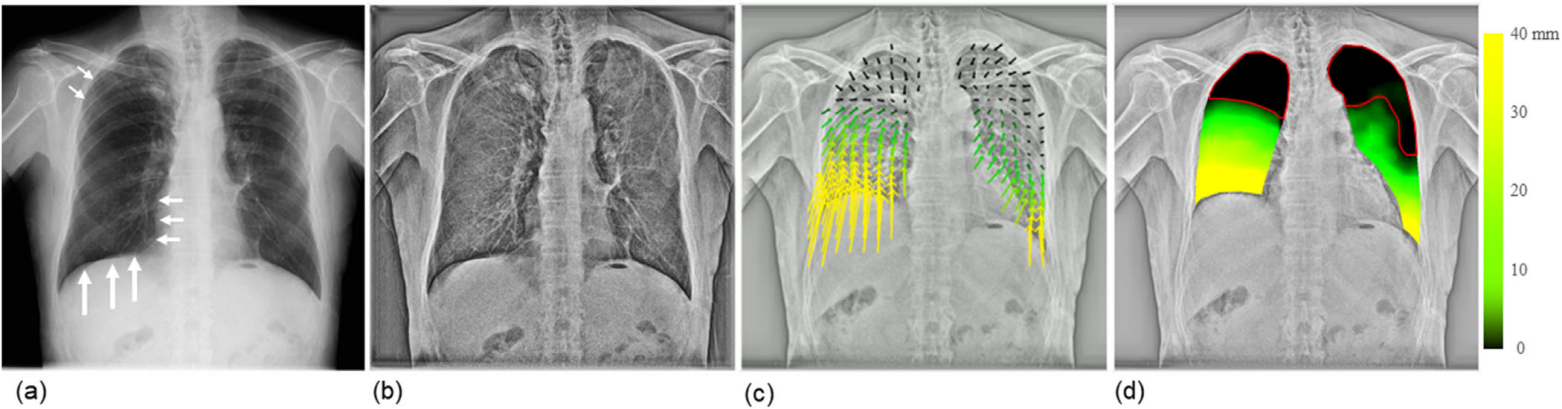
An example of a false‐negative case. Frame of the original dynamic chest radiography (DCR) images (a), bone‐suppressed DCR with enhanced high spatial frequency (b), vector‐map images (c), and displacement‐map image (d) of a 71‐year‐old man with right lung cancer (grade 2 pleural adhesion of the entire diaphragmatic surface). The patient showed poor motion in 34.4% and 45.9% of the right and left lung areas, respectively, a predicted VC of 90.5%, predicted FEV1 of 78.1%, FEV1/FVC ratio of 66.6, and predicted DLco of 41.6%. White arrows indicate areas with pleural adhesions; large, middle, and small arrows indicate strong, moderate, and mild adhesions, respectively. DLco, diffusing capacity of the lung for carbon monoxide; FEV1, forced expiratory volume in 1 s; FEV1/FVC, forced expiratory volume in 1 s/forced vital capacity; VC, vital capacity.
